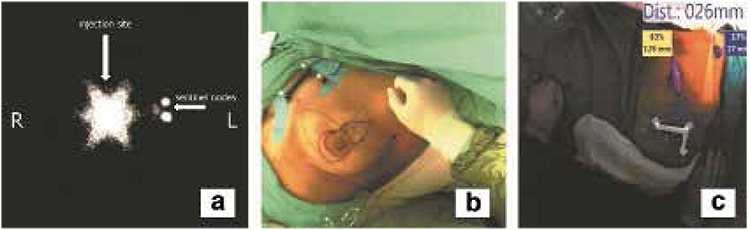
The lymphoscintigraphic image of the left breast on the left anterior oblique projection 30 min after radiotracer injection shows tracer accumulation in the injection site (long white arrow) and two adjacent axillary sentinel lymph nodes (short white arrow), b) The view of the operation theatre. Skin mark belonging to the sentinel lymph node is seen in the left axillary region, c) In the operating room, live video image shows two distinct radiotracer uptake foci in the injection site (rate: 83%, depth: 128 mm) and axillary region (rate: 17%, depth: 27 mm)
